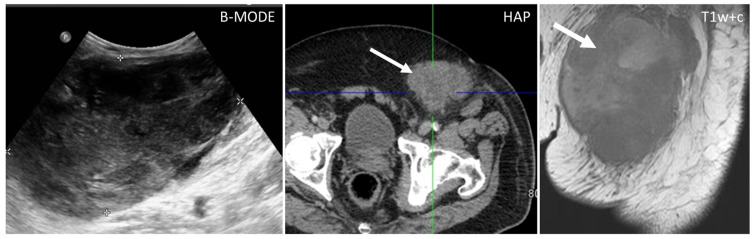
US, CT, and MR appearance of sarcomas of the abdominal wall in a 69-year-old male with a palpable mass. B-mode US (first picture) shows the presence of a large, heterogeneously hypoechoic mass, and anechoic areas corresponding to necrosis. Axial, contrast-enhanced CT scan on arterial phase (second picture) shows the presence of a poorly defined mass (arrow) with hazy borders and patchy enhancement. Sagittal, contrast-enhanced MR T1w image (third picture) shows the typical early diffuse enhancement of sarcomas.
